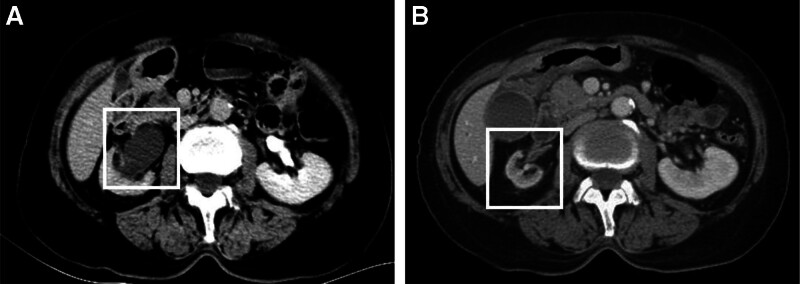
Imaging findings before and after ureteral stent placement. (A) Preplacement: CT urography (CTU) showing wall thickening and luminal narrowing of the right ureter at the level of the iliac vessels, with right hydronephrosis and hydroureter, as well as right renal atrophy with impaired function. (B) Postplacement: abdominal CT showing resolution of right hydronephrosis and hydroureter, along with right renal atrophy. CT = computed tomography, CTU = computed tomography urography.
